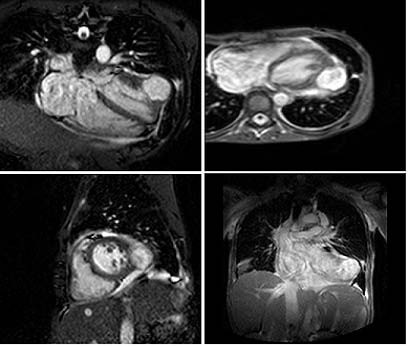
Cardiac magnetic resonance imaging. A 4.5 cm diameter left ventricular posterior wall abscess contained by only a 2 mm thin layer of myocardium.
